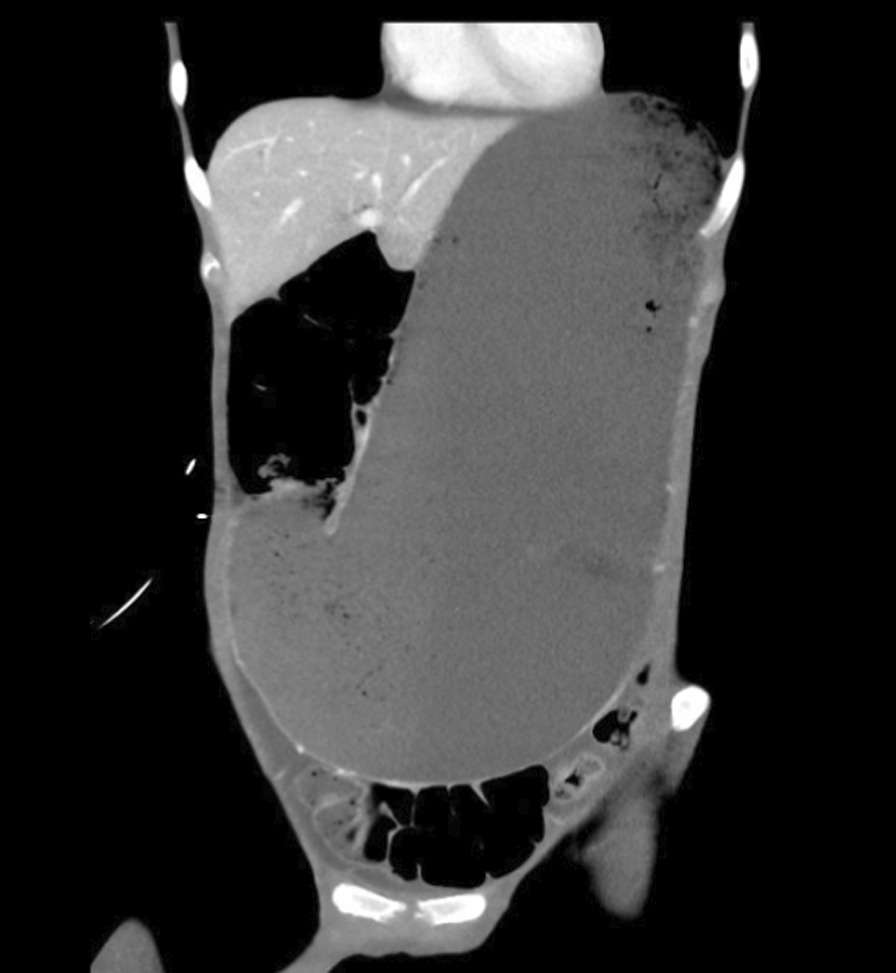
Computed tomography coronal view
